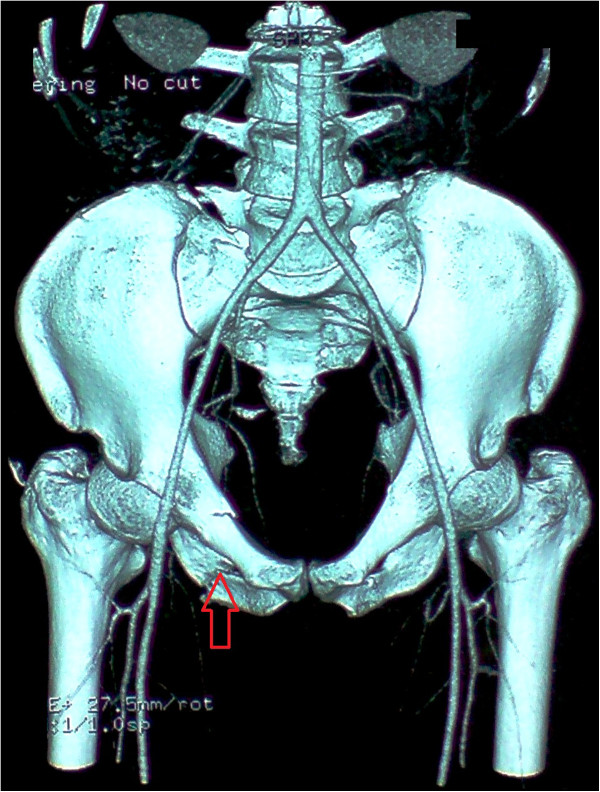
Computed tomography (CT) showing a right ischio pubic rami fracture.
